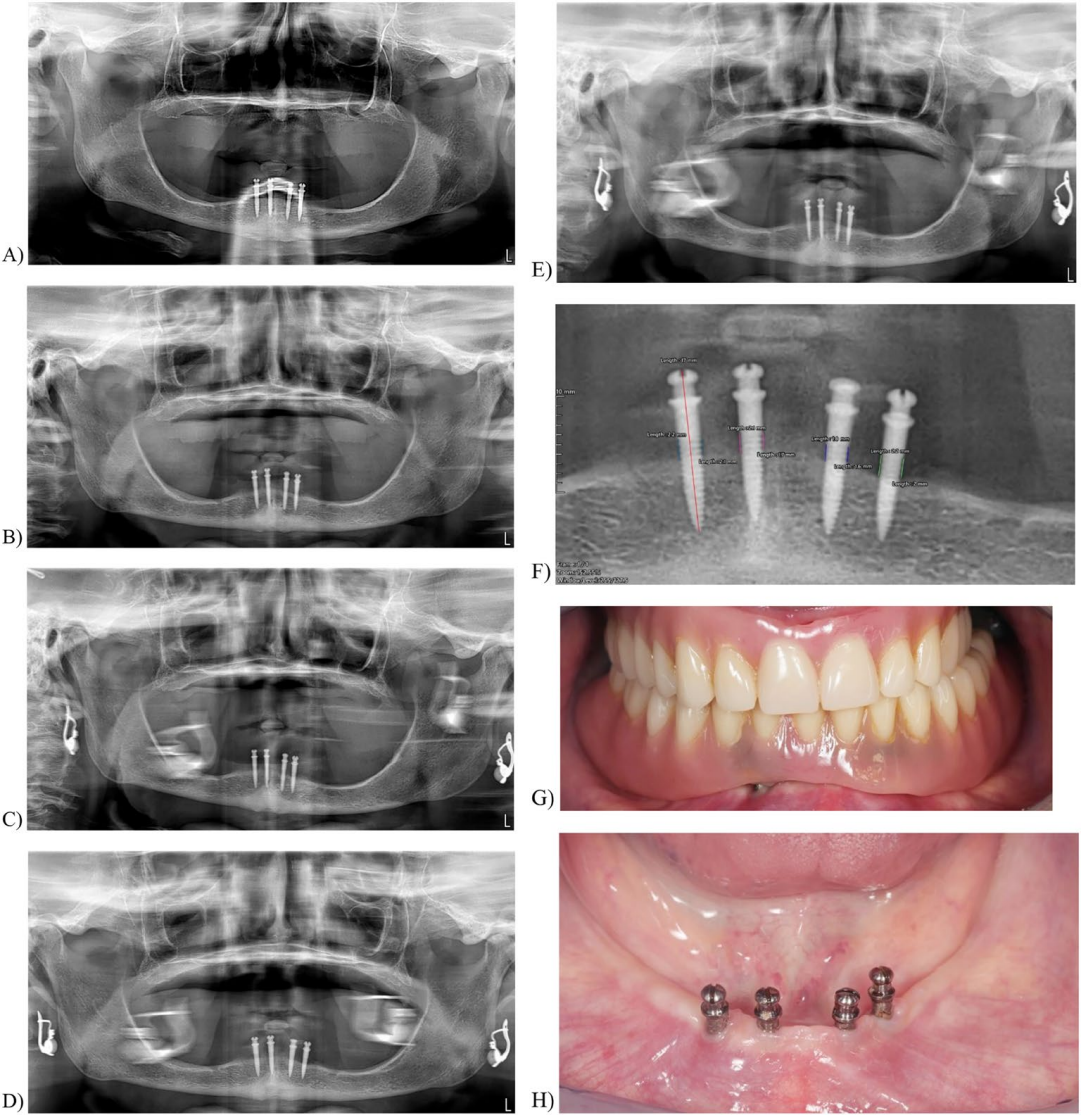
Example of a edentulous patient treated with four Dentatus Atlas Implant System. (A) Orthopantomography (OPG) showing the T0. (B) Orthopantomography (OPG) showing the T1. (C) Orthopantomography (OPG) showing the T5 (D) Orthopantomography (OPG) showing the T8 (E) Orthopantomography (OPG) showing the T10 (F) MBL calibration with Weasis Software (G, H) Clinical results.
